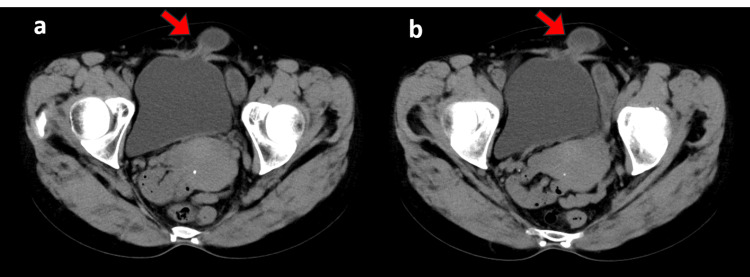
CT scan of the abdomen (axial). a, b: The abdominal incisional hernia in the left lower abdomen and a prolapsed bladder from the same area. The hernia portal was about 1.3 cm.
